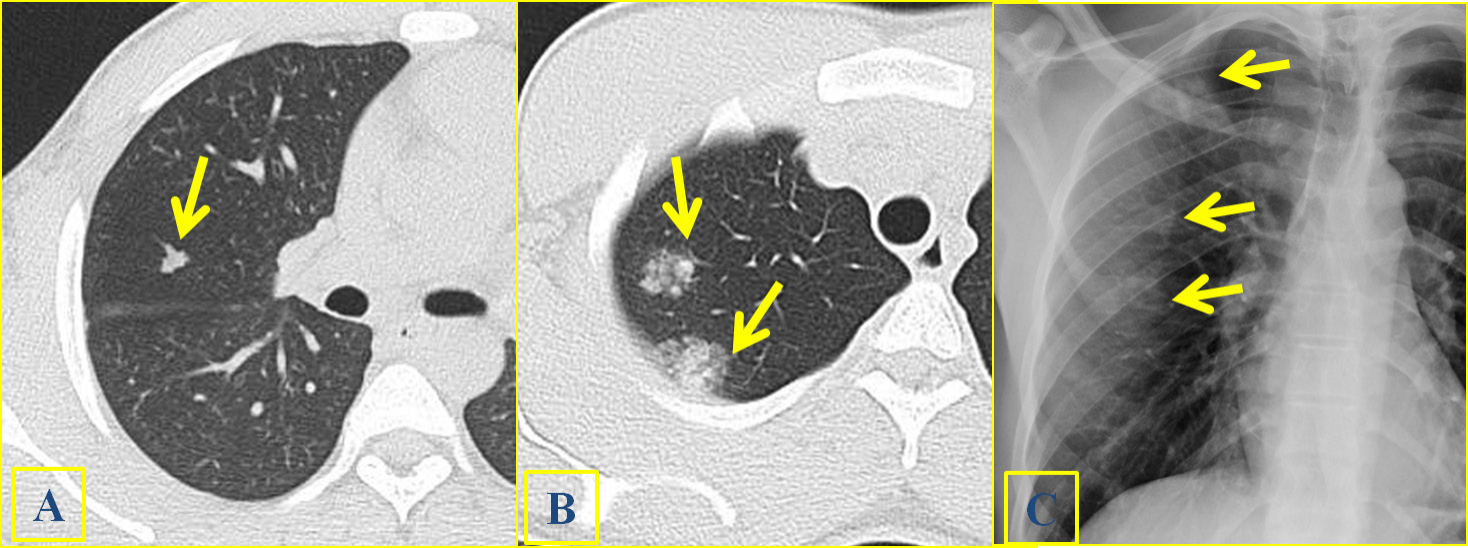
A 25-year-old male with clinical suspect of schistosomiasis and absence of pulmonary clinical findings. Multiple nodules (arrows) were identified at HRCT, including a solid lobulated nodule (2A) and two contiguous mixed nodules with peri-nodular halo on the upper lobe (2B). Solid and mixed nodules (arrows) can also be easily identified on CR (2C). The diagnosis of schistosomiasis was achieved by means of CT guided biopsy.
